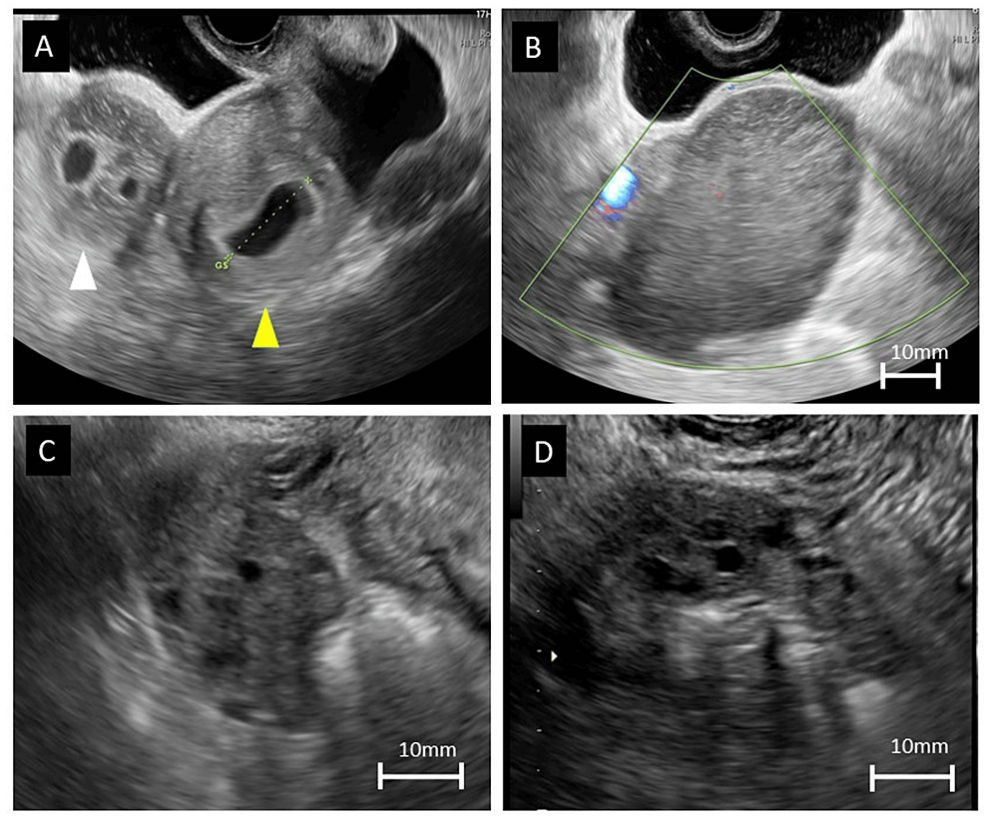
Transvaginal ultrasound imaging and the time course of ovarian changes A: Image of the preoperative ovary. Enlargement of the right ovary can be observed (white arrowhead), whereas the gestational sac is detected in the uterine cavity (yellow arrowhead). The right ovary measures 93x70 mm. B: Image of the preoperative ovary. Color Doppler imaging of the right ovary shows no abnormal blood flow. C: Image of the ovary at the one-week follow-up after discharge. The right ovary measures 37x24 mm. D: Image of the ovary at one month after delivery. The right ovary measures 23x17 mm.
